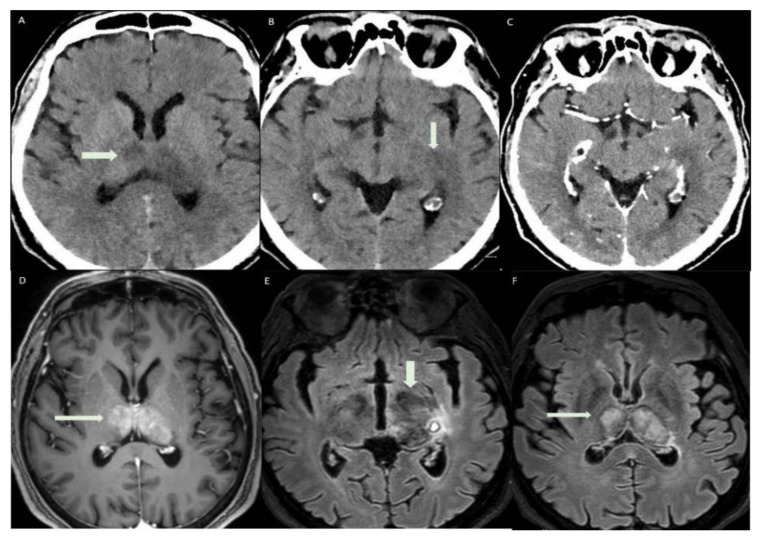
(A–C) Axial CT images show regular densitometric evolution of ischemic areas, with less hypodensity and reduction in hemorrhagic focus in the left parahippocampal region. (D) Axial TSE T1-weighted post contrast sequence documents reperfusion phenomena in both thalami (arrow); In (E,F), axial FLAIR-weighted sequences show a reduction in the diameter of hemorrhagic focus, compared to the first MRI exam, and less intense signal in the ischemic foci.
